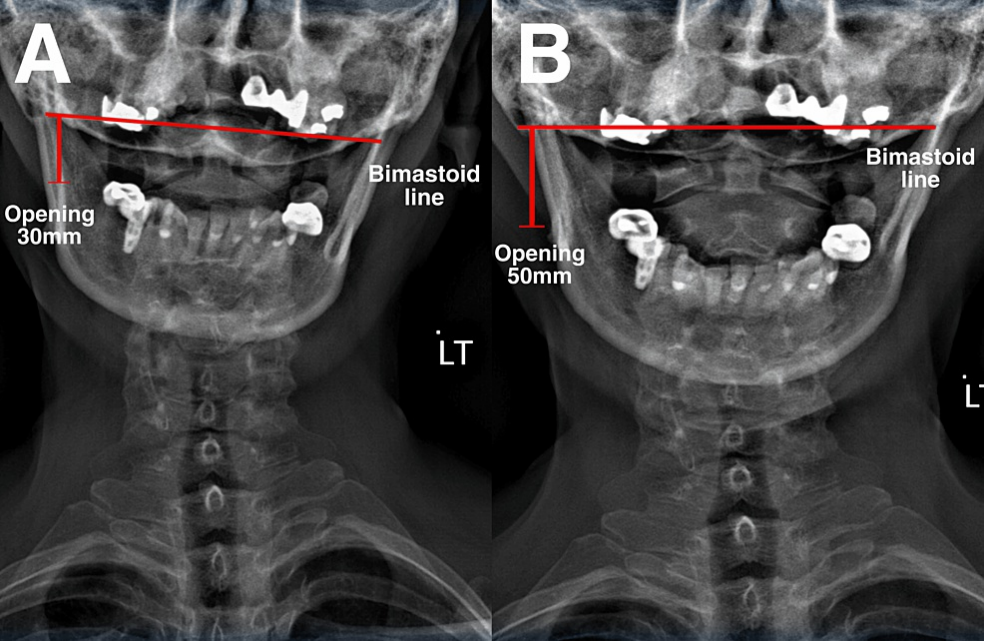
Anterior posterior open-mouth radiographs before and after therapy. A) Pre-treatment. Unbalanced bimastoid line and MMO of 30 mm; B) Post-treatment. Balanced bimastoid line and MMO of 50 mm. MMO: Maximum mouth opening.
